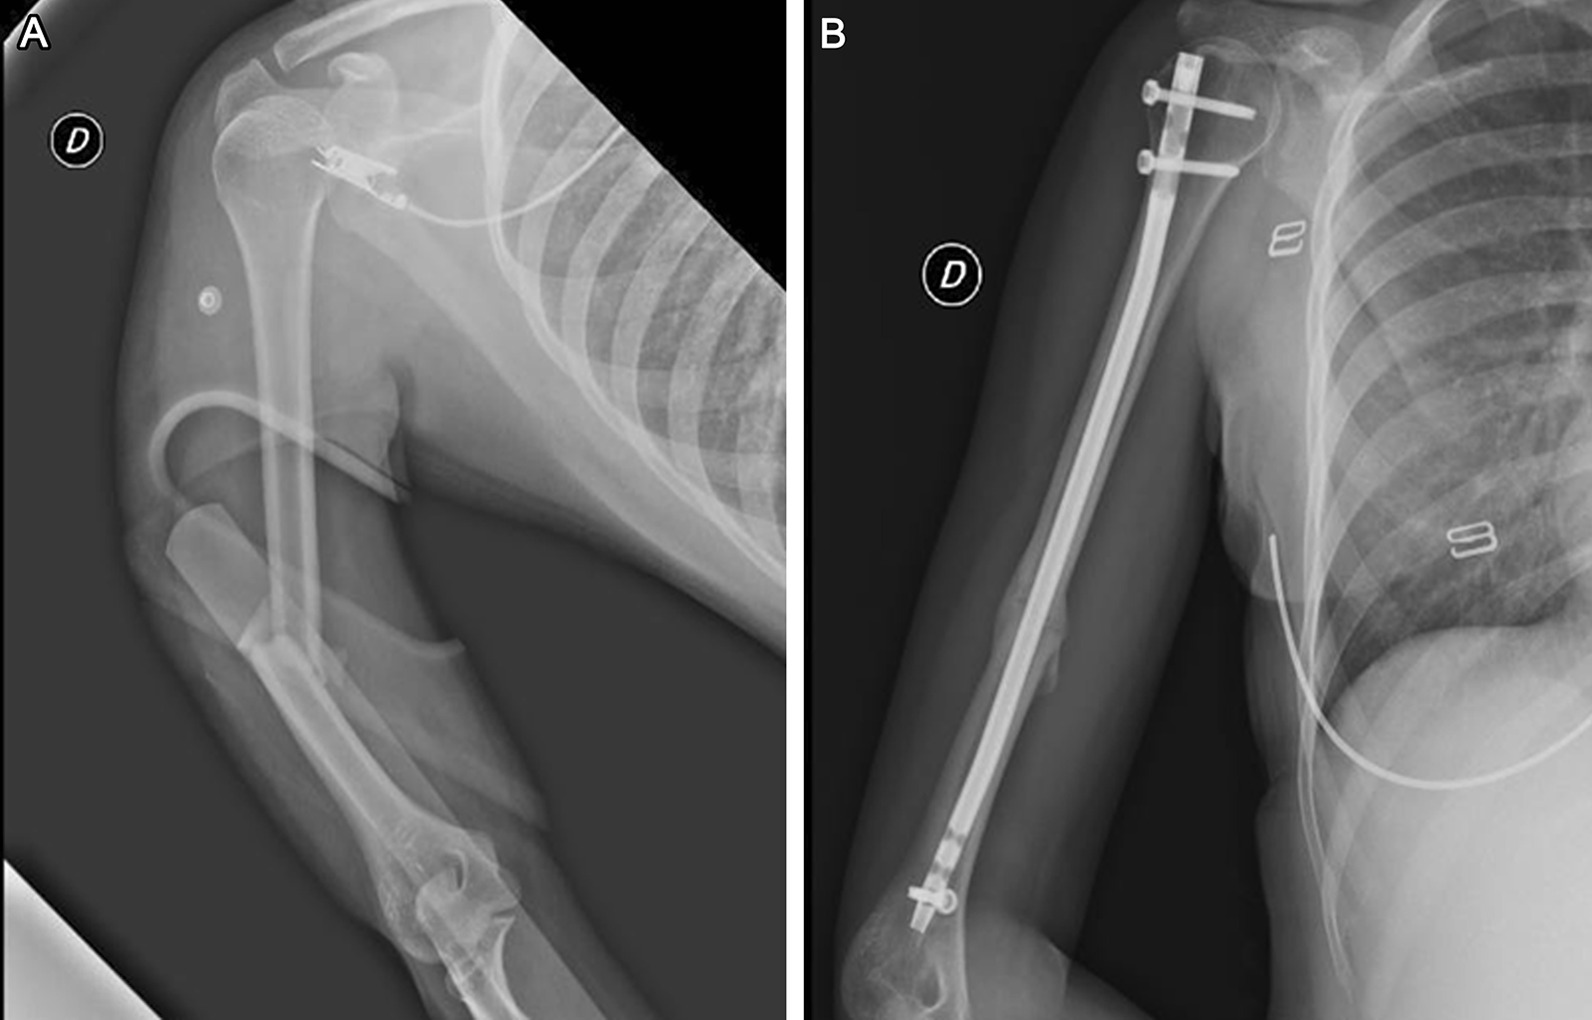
A Radiograph shows a fracture of humeral diaphyseal segment with a wedge fragment (AO cl. 12B2). B Good fracture healing at 180 days post-op after osteosynthesis with a curvilinear design intramedullary nail
